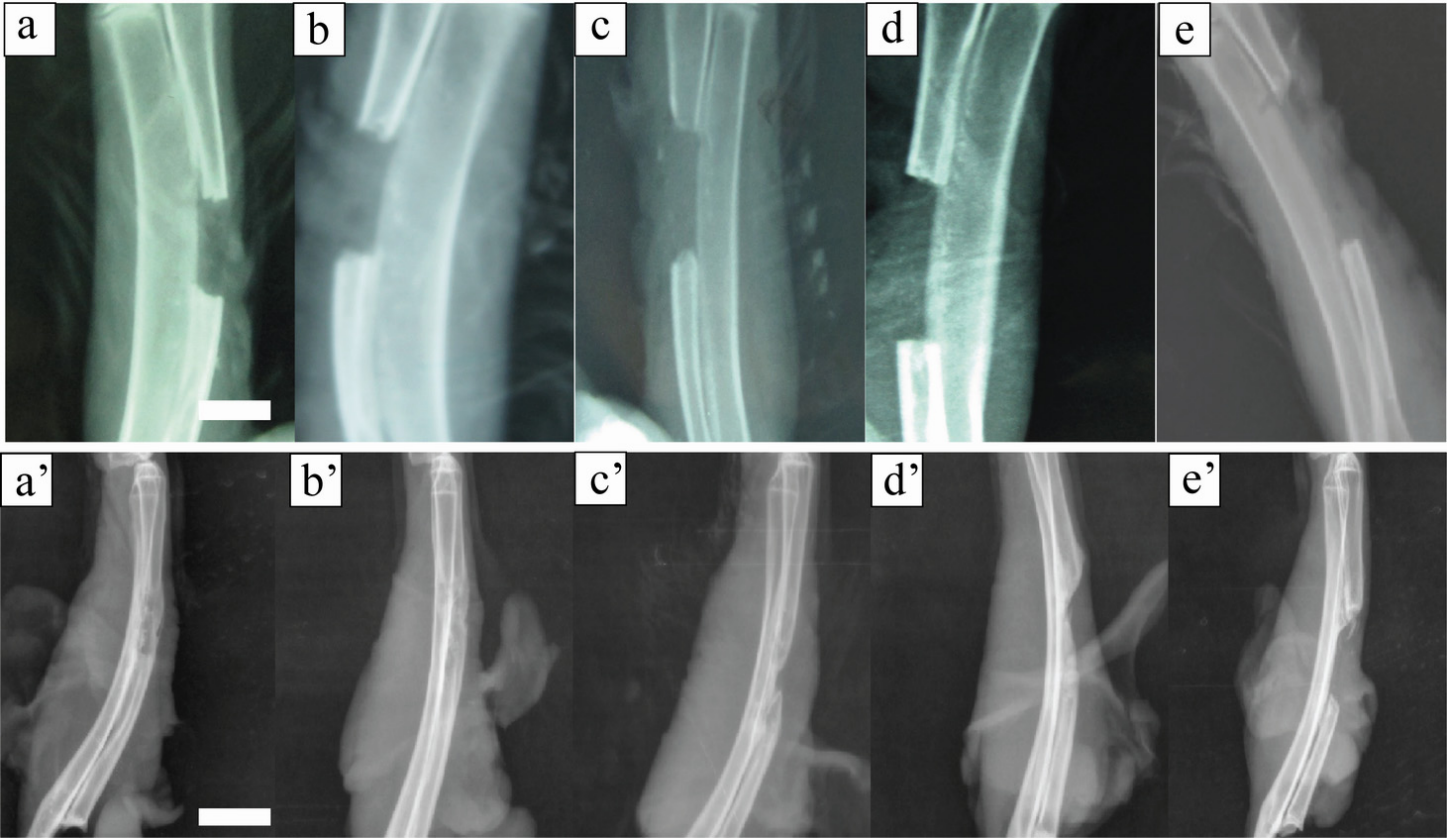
Radiograph of different radius defects just after operation and 12 weeks postoperatively. The size of the central bone defect gradually increased with respect to an increase in created defect size (a,a’: 1.0cm, b,b’: 1.2cm, c,c’: 1.4cm, d,d’: 1.7cm, e,e’: 2.0 cm, a-e: just after operation, a’-e’: 12 weeks postoperative, scale bar: 10mm).
